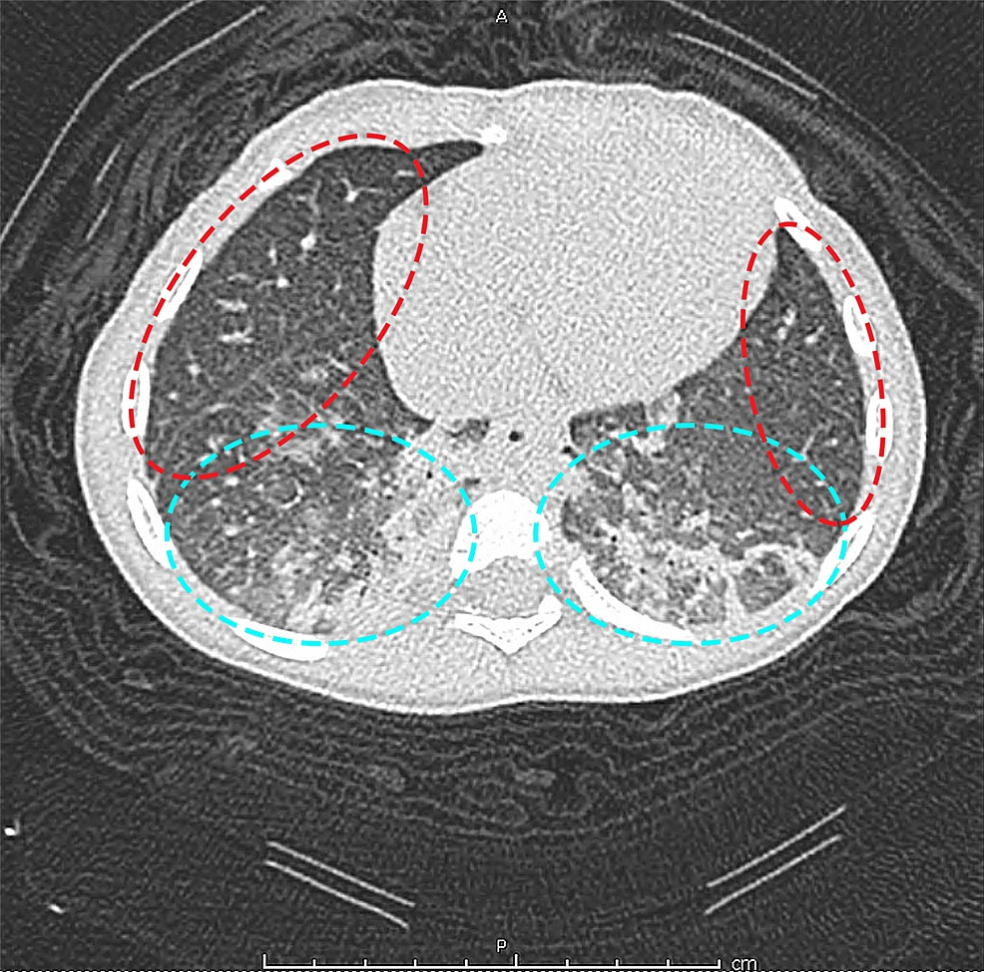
Chest CT without contrast showing bilateral dependent airspace opacities (circled in bright blue) with peripheral groundglass opacity and high-density material (circled in red) consistent with pulmonary hemorrhage
